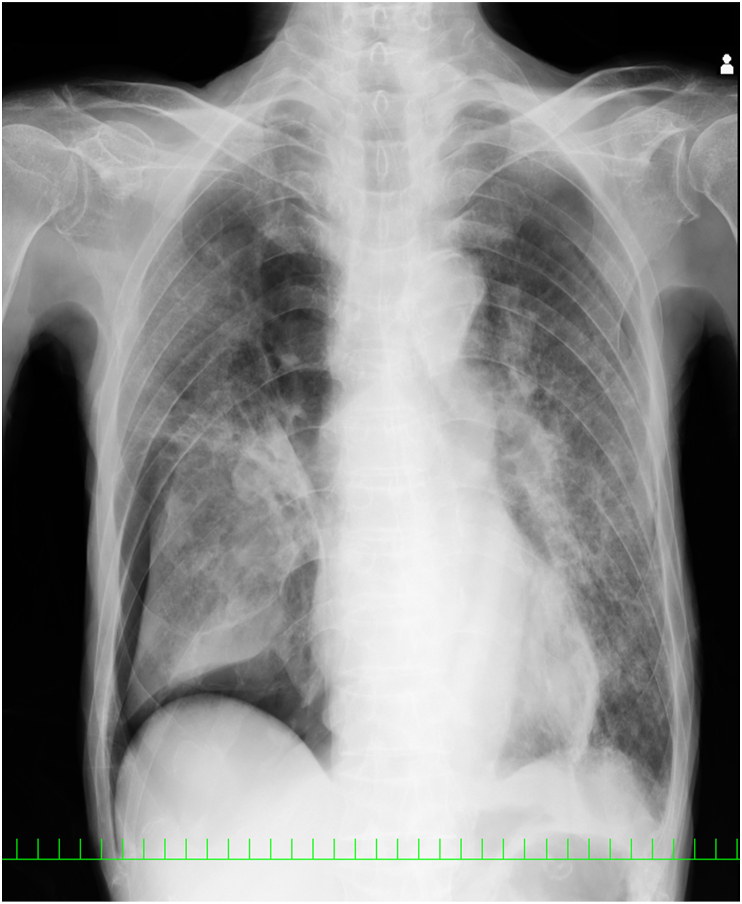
Chest radiograph of pneumothorax in coronavirus disease 2019 (patient 1).
